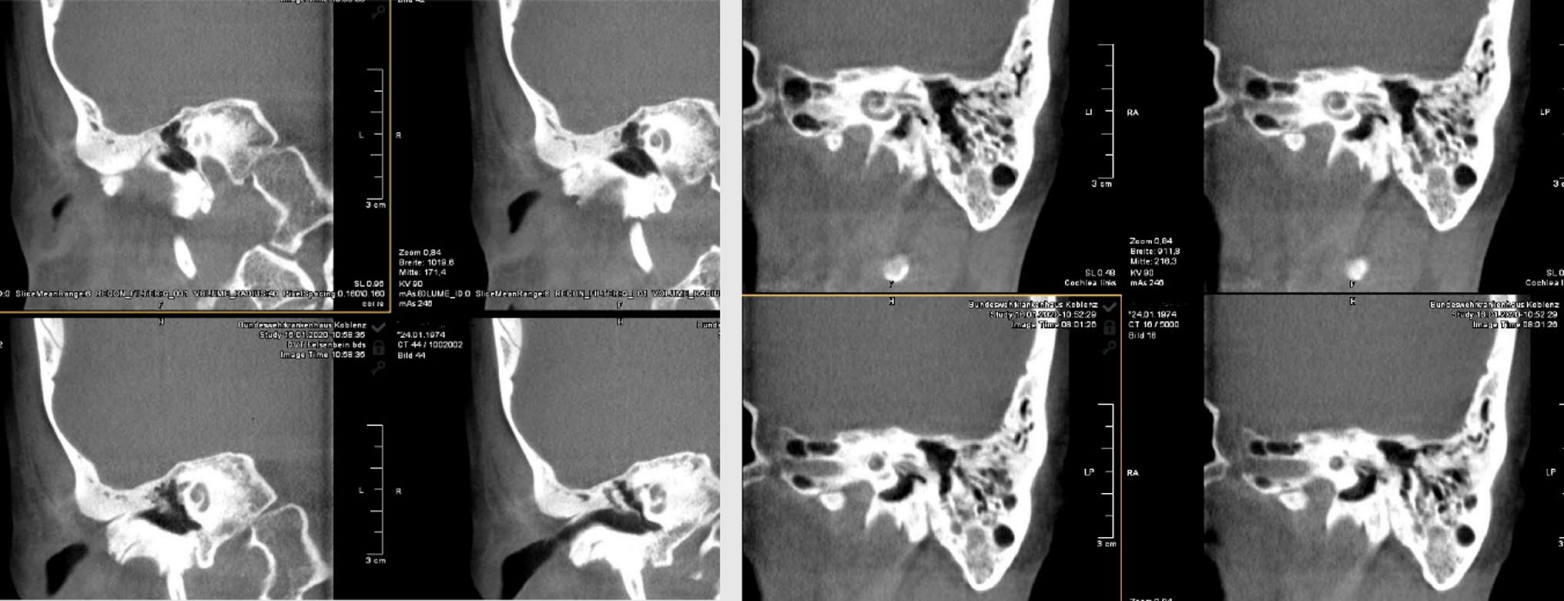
CBCT of the petrous bone of patient 2 (as an example of the surgical procedures in patients 1 and 2)
